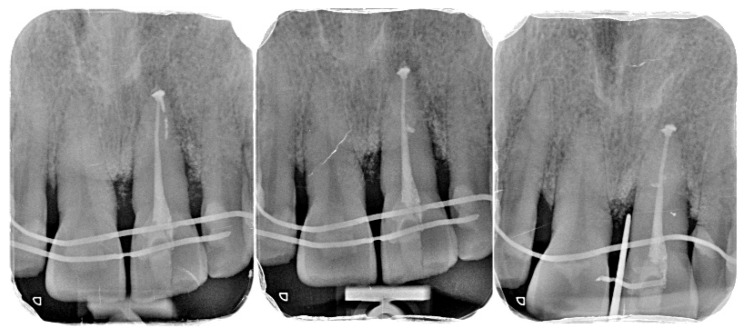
Standardized periapical radiograph, endodontic day and 24 weeks later.
